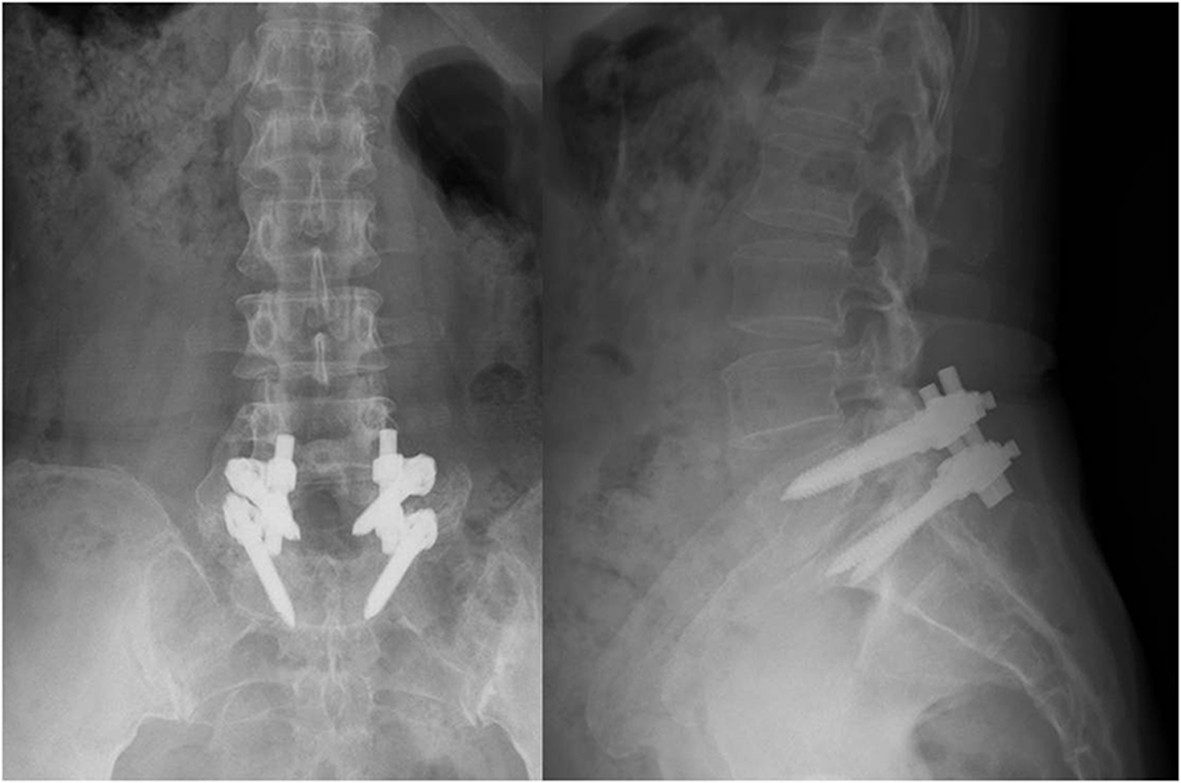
Example of solid fusion after surgery. A 55-year-old female underwent L5 laminectomy and L5-S1 instrumented posterolateral fusion. Two-year postoperative radiographs revealed fused L5-S1 segments and well-maintained implants.
